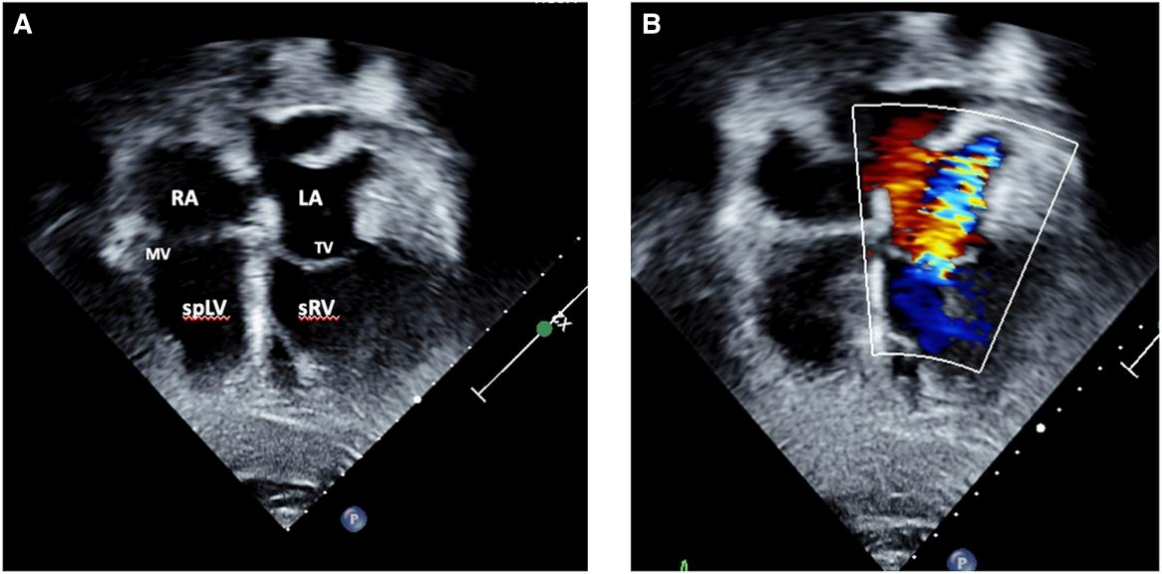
Transthoracic echocardiography of a 63-year-old male presenting with ACS and incidental findings of *(A)* atrio-ventricular and ventriculo-arterial discordance in the setting of ccTGA with pulmonary stenosis, VSD as well as moderate regurgitation of the systemic AV-valve *(B)*. LA, left atrium; MV, mitral valve; spLV, sub pulmonary left ventricle; sRV, systemic right ventricle, TV, tricuspid valve.
